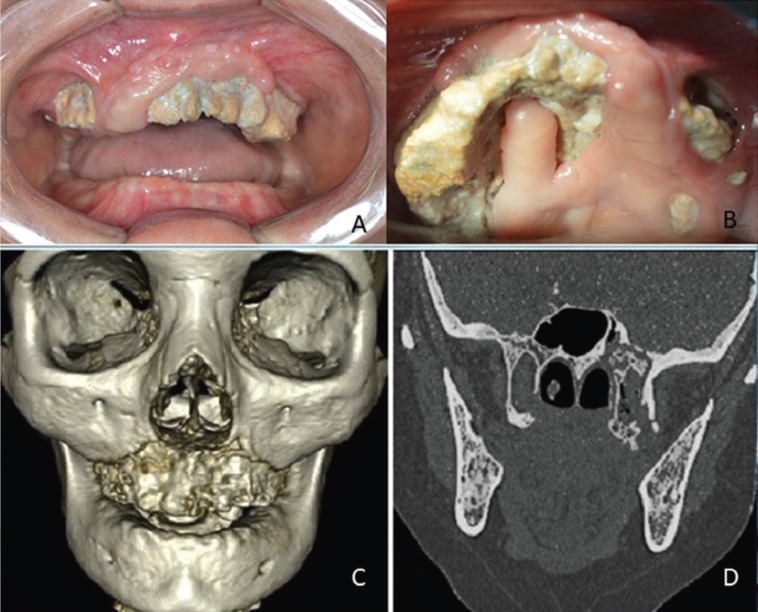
A: Intra oral clinical picture of extensive exposed necrotic bone. B: Palatine view showing exposed necrotic bone. C: Three-dimensional reconstruction CT-scan showing necrotic area compromising the whole maxilla. D: Coronal bone window image showing necrotic bone extended toward the skull base.
